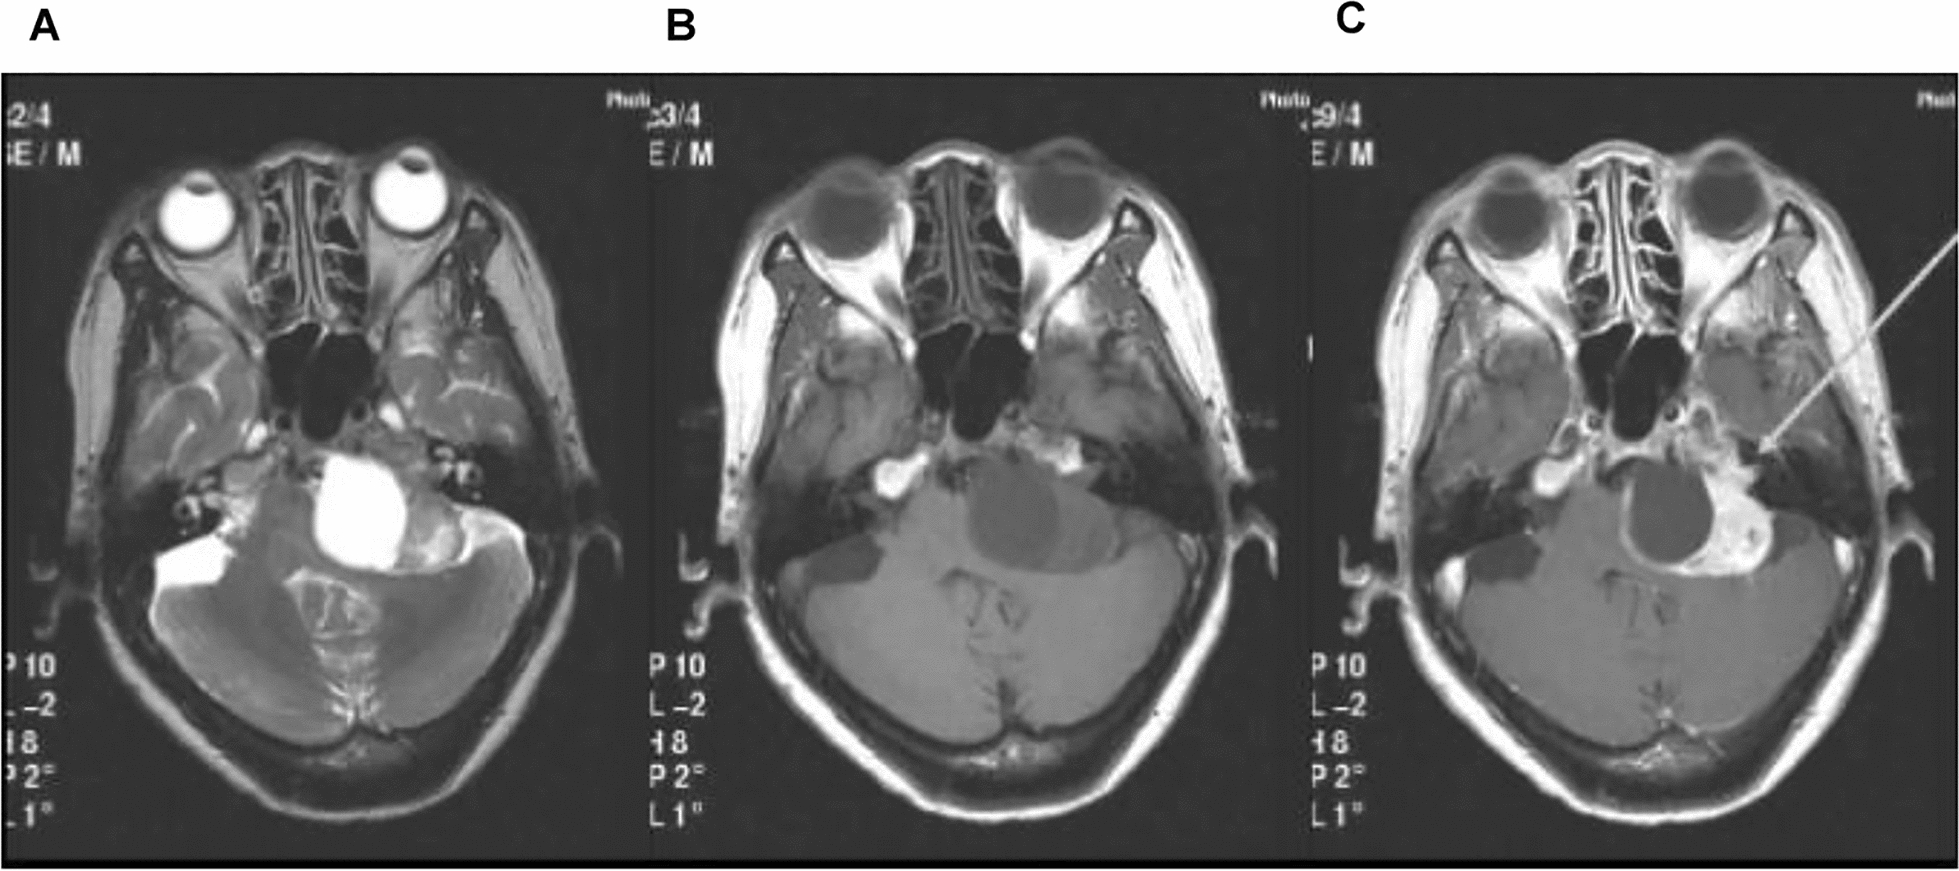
MRI scanning with VS of left cerebellopontine angle: a T1-weighted MRI shows the hemorrhage as an isointense area. b MRI depicts hemorrhage as a hypointense area on the T2-weighted images. c T1-weighted MRI with contrast demonstrates heterogeneous enhancement of VS
